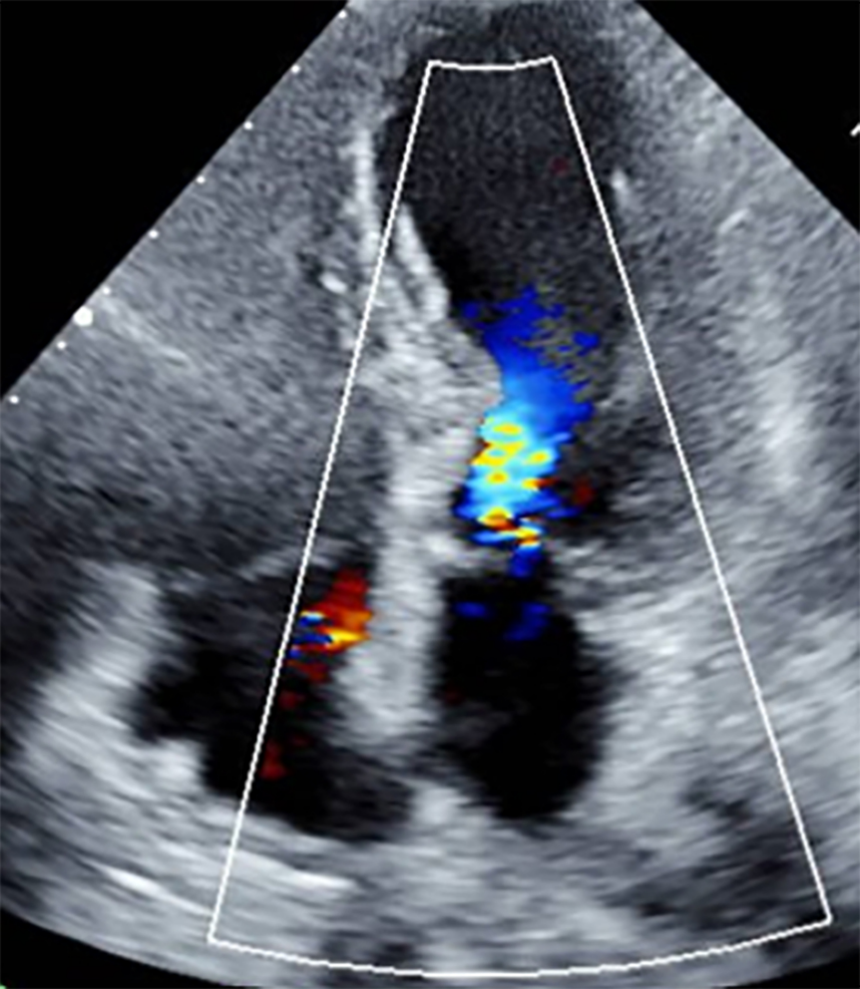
Apical four chamber view using handheld POCUS. Apical
